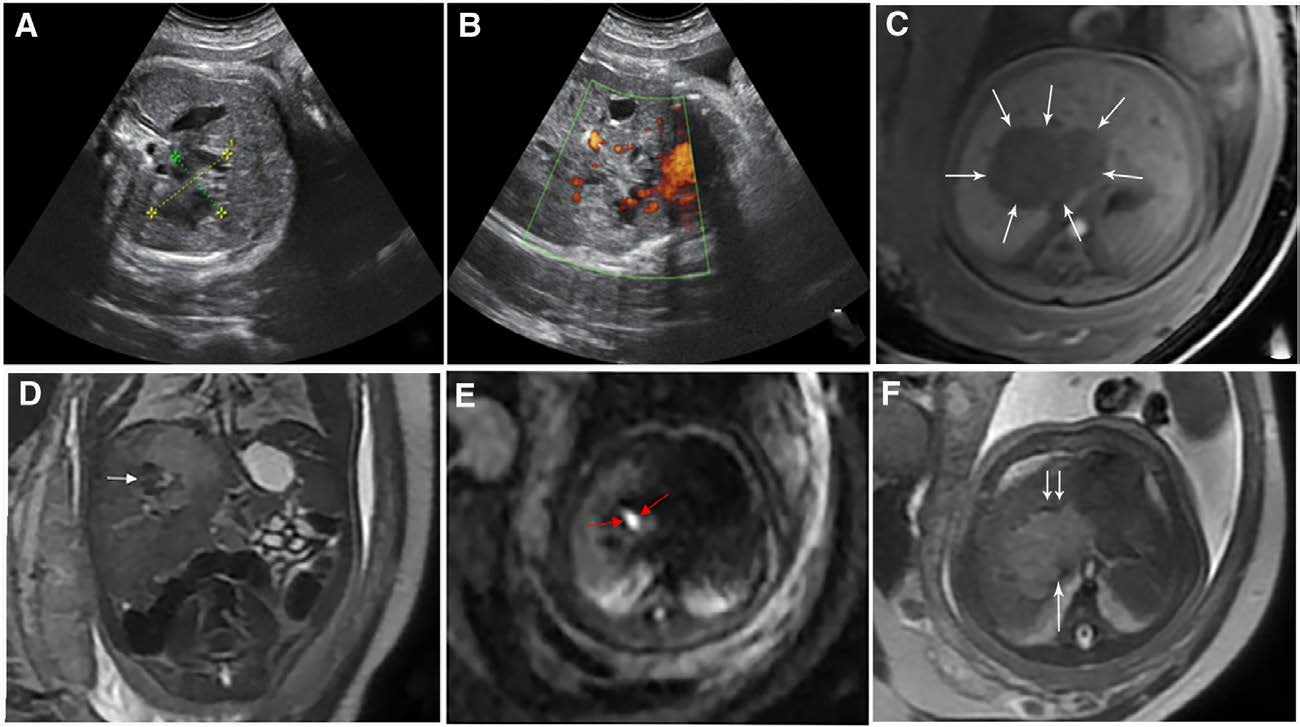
Prenatal ultrasonography and magnetic resonance imaging (MRI) findings. (A) Ultrasonography showing the presence of a continuous-space lesion measuring 4.4 cm × 3.2 cm with mixed echo in the liver. (B) CDFI detected punctuated blood flow in the mass and a short bar blood flow signal around the nodule. (C) *T*1-weighted axial MRI showing a well-defined lesion (arrow). (D) *T*2-weighted coronal MRI demonstrating hypointense in the hyperintense area (arrow). (E) Diffusion-weighted imaging revealing minimal diffusion restriction and partial diffusion restriction (red arrow). (F) *T*1-weighted axial MRI showing empty vessels around the mass (double arrows) and the inferior vena cava deformation next to the mass (long arrow).
